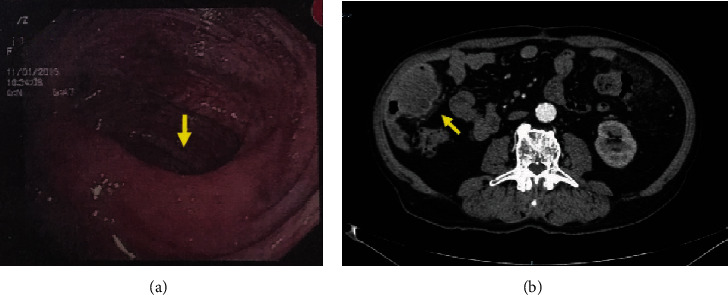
Endoscopic imaging of lung adenocarcinoma metastasis in the splenic flexure and (a) CT imaging of left colonic wall thickening (b).
